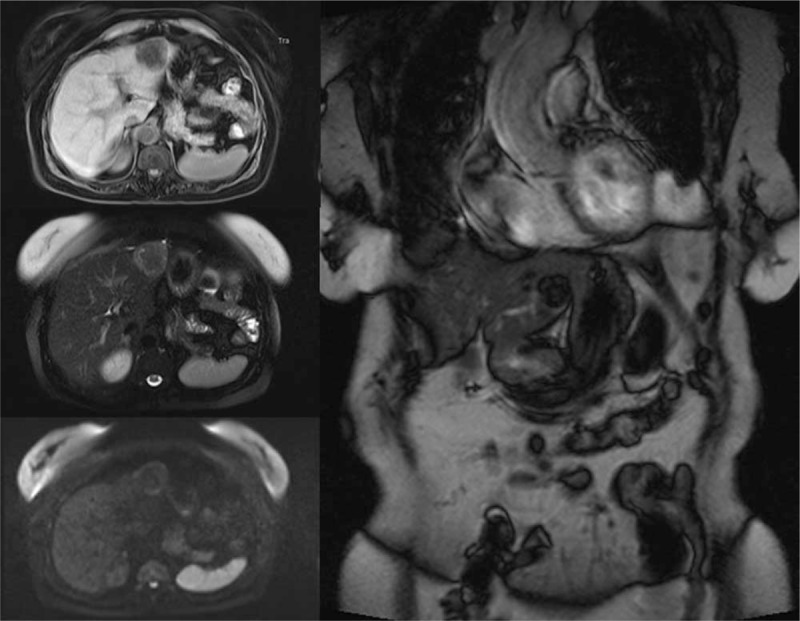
The MRI reported that a nearly-circular abnormal signal shadow, about 4.4×4.2 cm^2^ in size, was seen in hepatic left lateral, presenting a lamellar high signal in phase, a low signal out phase, and an equal or low signal in T2 fat-suppressed imaging, which is considered liver cancer with steatosis. MRI = magnetic resonance imaging.
